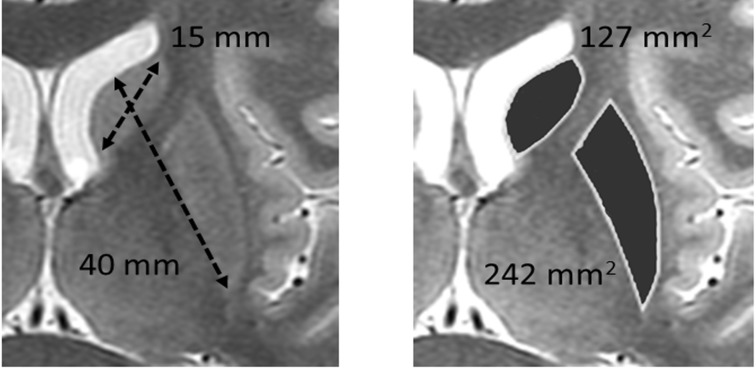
Striatal size measured on magnetic resonance images; major and minor axes are 40 and 15 mm, respectively; putamen and caudate areas are 242 and 127 mm^2^, respectively
